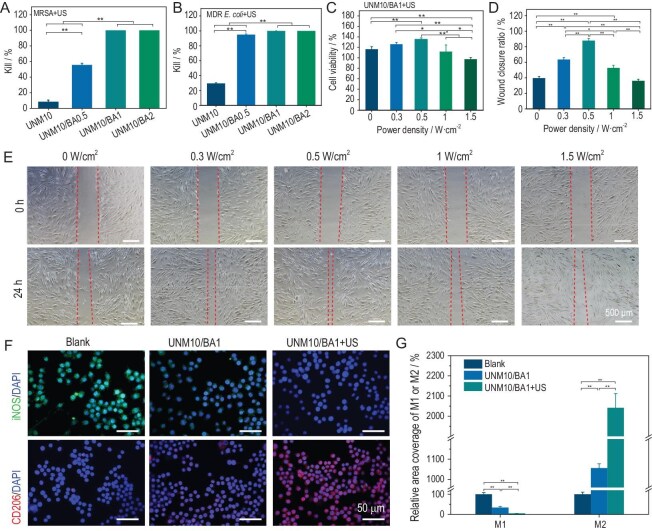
Sonopiezoelectric-effect-mediated regulatory effects of UNMx/BAy. (A and B) Bactericidal ratios of hydrogels against MRSA (A) and MDR *E. coli* (B) with 1.5 W/cm² ultrasound treatment. (C) Cell viability under exogenous ultrasound-mediated UNMx/BAy treatment. (D and E) Wound closure ratio (D) and representative images (E) of cell scratches at 24 hours post-treatment with hydrogels mediated by ultrasound at different power densities. (F and G) Representative images (F) and relative expression levels (G) of M1 macrophages and M2 macrophages under different treatment conditions. P* < 0.05, *P* < 0.01.
